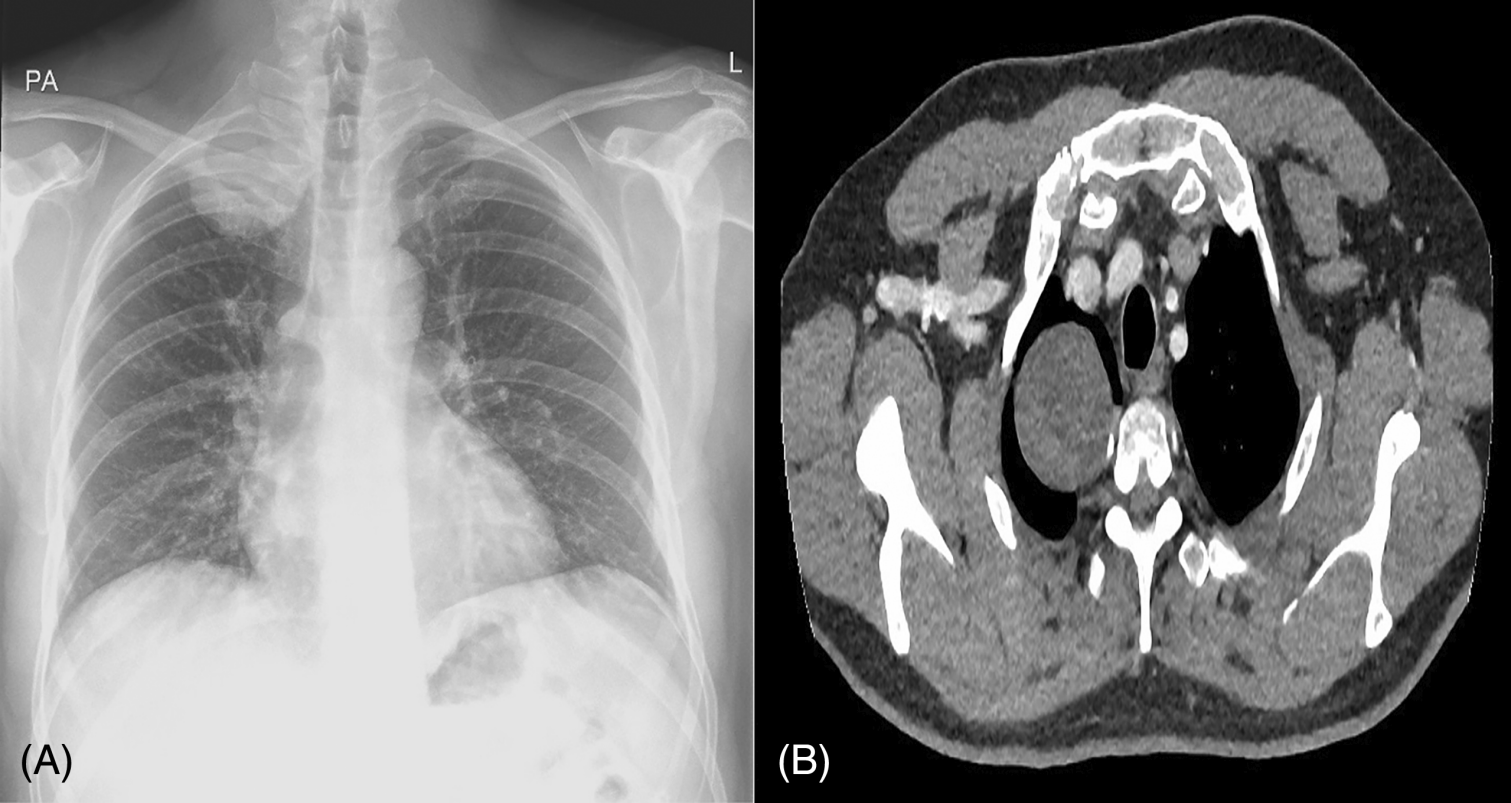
(A) Chest radiograph demonstrating a well‐defined 6 cm opacity in the right apex. (B) CT thorax (axial section) demonstrating a 5 cm × 5 cm well circumscribed mass in the right apex. The mass is homogenous and hypodense with a mean density of 18.98 HU which would be typical of intrathoracic neurogenic tumours, like a Schwannoma. It is inseparable from the chest wall.
